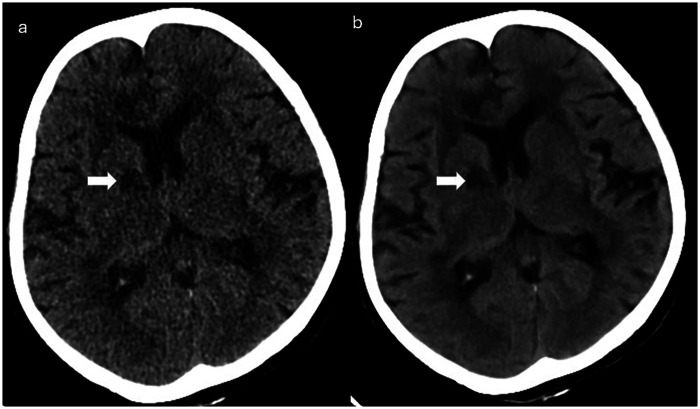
Axial CT LD images of an 80-year-old woman with a right lentiform nucleus acute infarct. The small hypoattenuation area (arrows) was clearly delineated in DLIR (b) while preserving image noise compared with iDose^4^
